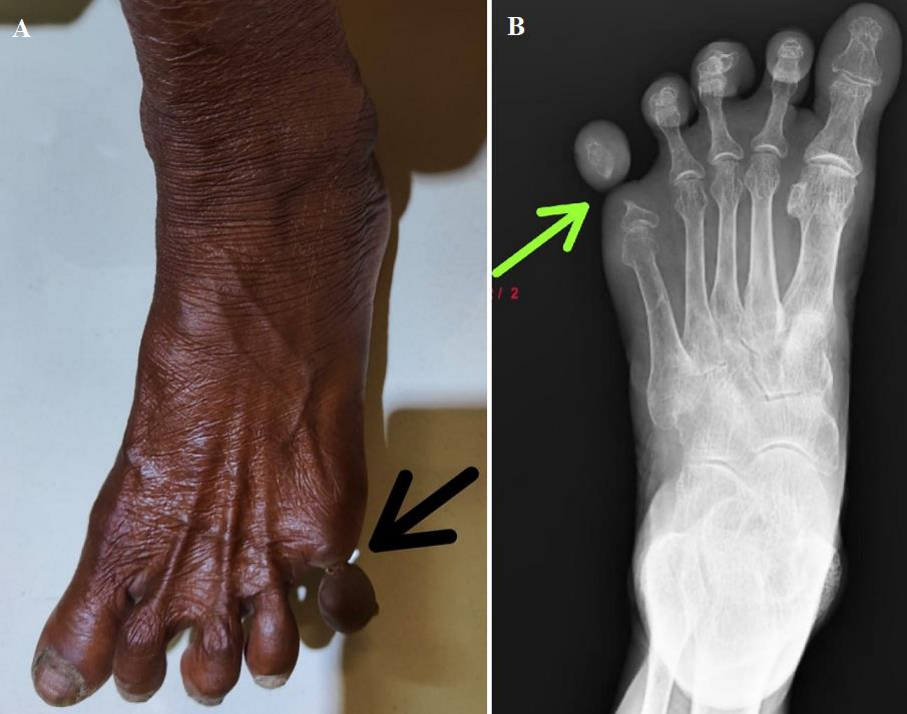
A) “ainhum”; B) X-ray of left foot
